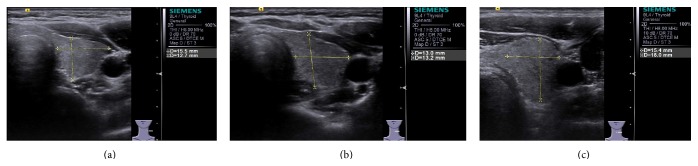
Two-dimensional ultrasound images of (a) normal thyroid, (b) GD thyroid, and (c) HT thyroid.
